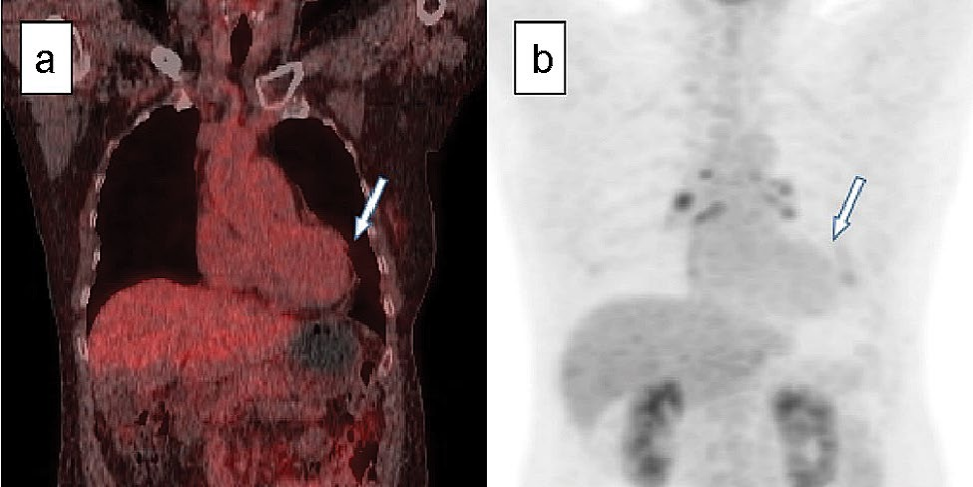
Follow-up ^18^F-FDG-PET/CT image. (a) A repeat ^18^F-FDG-PET/CT scan was performed after 3 months of glucocorticoid treatment, and the pericardial inflammation markedly improved with a decreased ^18^F-FDG uptake (SUVmax = 1.5) in the pericardium (arrow). (b) ^18^F-FDG uptake in the pericardium (arrow) in PET image. ^18^F-FDG-PET/CT: fluorine-18 fluorodeoxyglucose -positron emission tomography/computed tomography, SUVmax: maximum standardized uptake value
